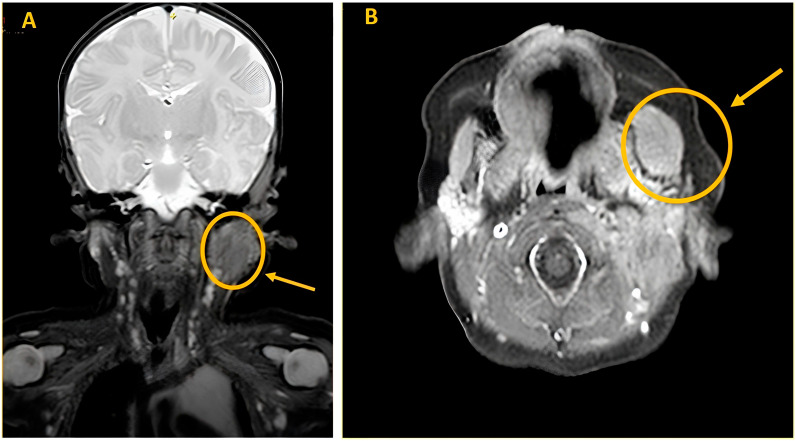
Head and neck MRI. Examination performed before and after intravenous bolus administration of 1 ml of paramagnetic contrast medium, such as Dotarem (gadoteric acid) 0.5 mmol/mL. (A) Coronal image T2 dixon: Fusiform dilation of the left masseter muscle (20 mm × 15 mm × 21 mm) with minimal, homogeneous hyperintensity in T2 signal. Fusiform swelling at the level of the cranial slope of the left sternocleidomastoid muscle (13 mm × 14 mm × 31 mm). (B) Intravenous contrast-enhanced axial image T1 dixon: Homogeneous isointensity of the signal in T1 with no perifocal edema or pathological enhancements after contrast administration.
